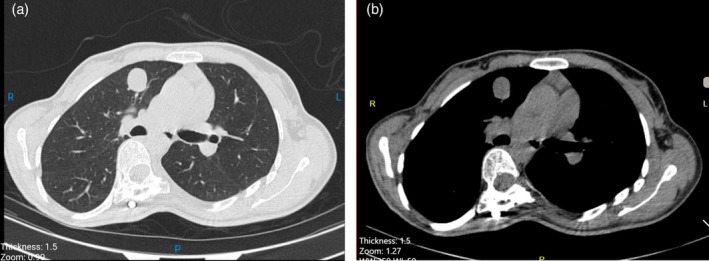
(a) Axial chest CT (parenchymal window) showing a well‐circumscribed 3 cm round lesion in the right upper lobe. (b) Mediastinal window of the same lesion showing no visible fat density or popcorn calcification.
